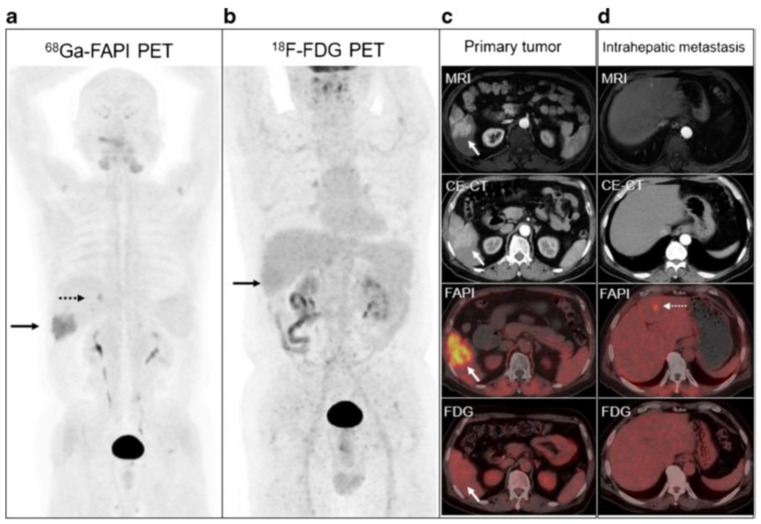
Patient with hepatitis B virus infection for initial staging of moderately differentiated HCC. ^68^Ga FAPI-04 (a), [^18^F]-FDG PET/CT (b), and MRI and contrast-enhanced CT (CE-CT) (c) concordantly showed the primary lesion at the right lower lobe (solid arrows). An extra 0.9-cm metastasis at the left internal lobe (dotted arrows) confirmed on ^68^Ga FAPI-04 PET/CT and liver MRI was not shown on CE-CT or [^18^F]-FDG PET/CT (d). (images reproduced with permission from [70]).
